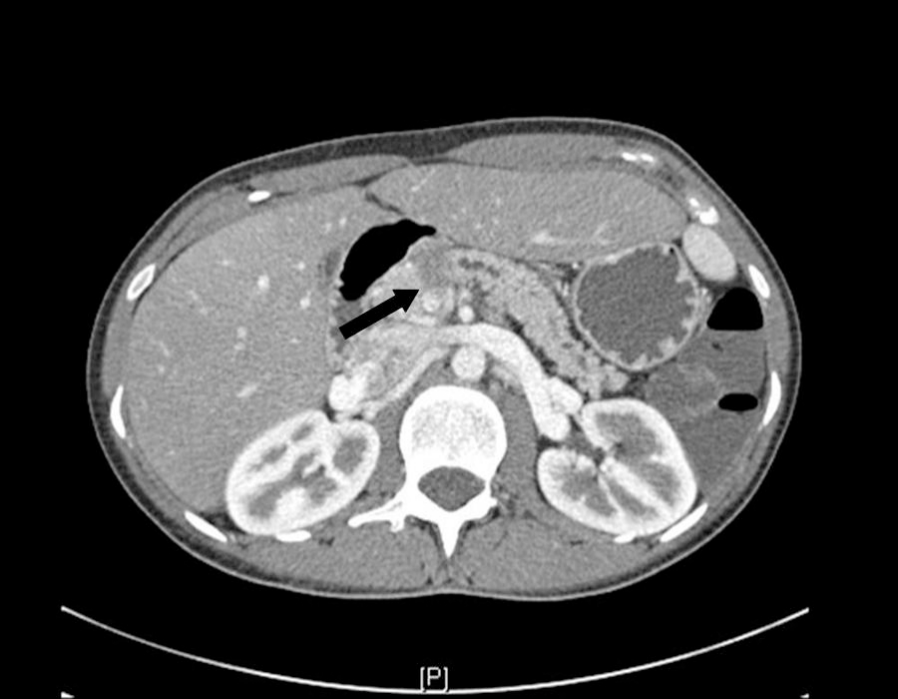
CT scan of abdomen with pancreatic mass (arrow).
